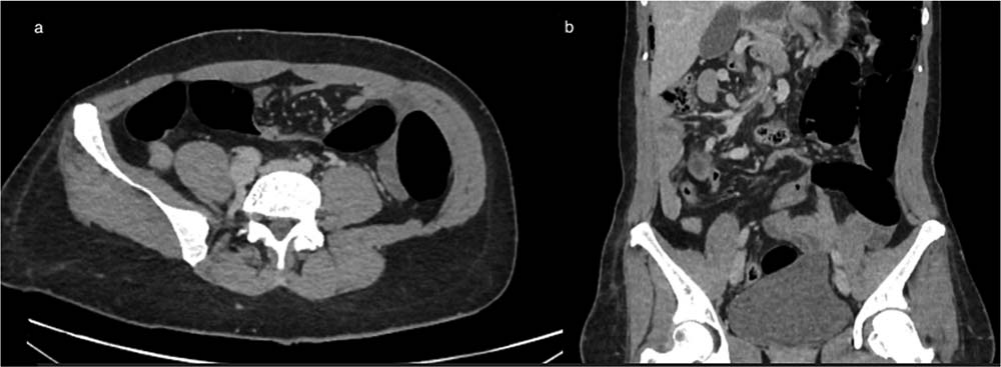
(a) Axial CT showing marked colonic distension upstream with an abrupt transition point in the right abdomen, consistent with mechanical obstruction related to volvulus (b) coronal CT reconstruction demonstrating colonic dilation and the corresponding transition zone.
